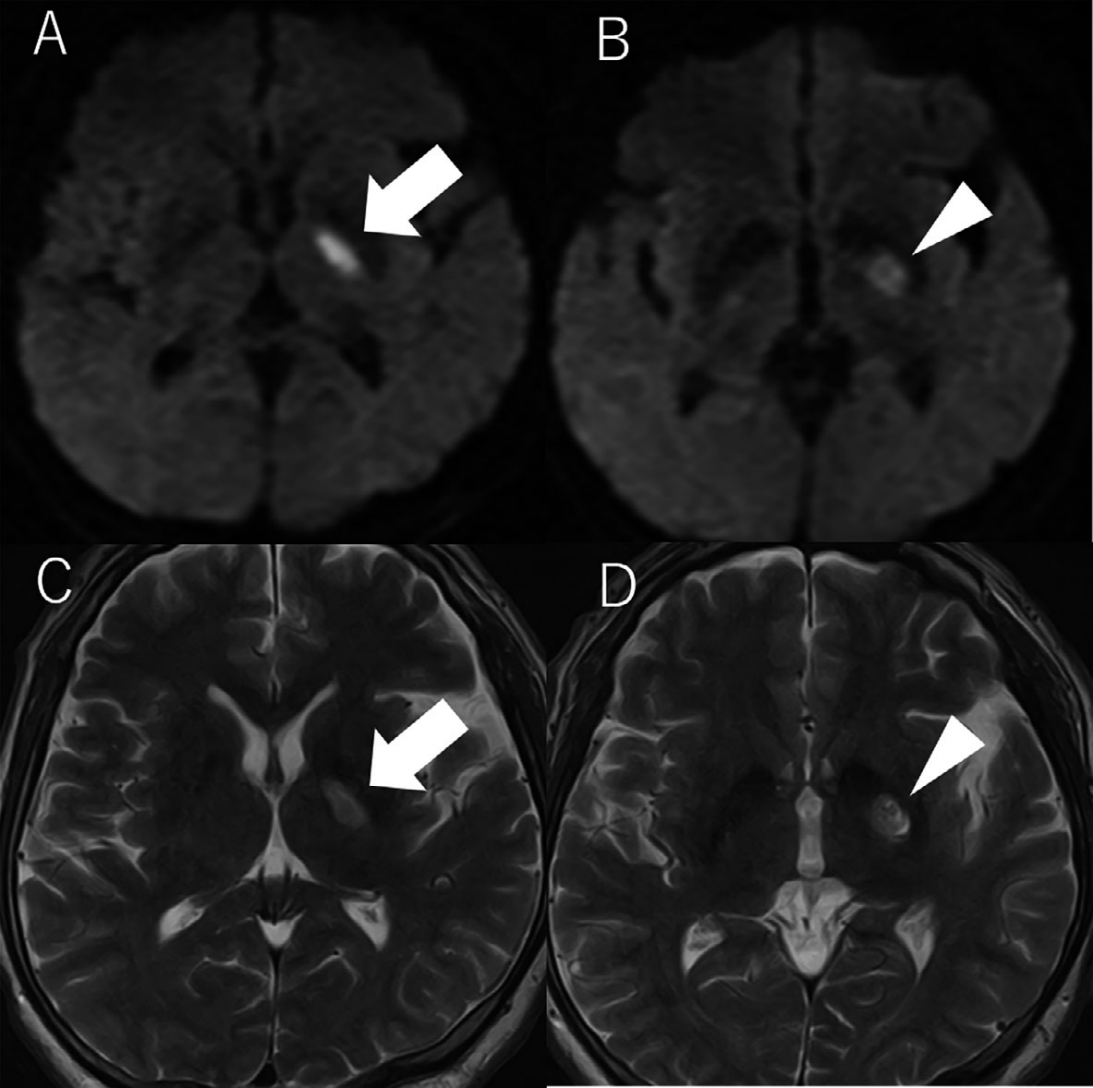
Diffusion‐weighted (A and B) and T2‐weighted (C and D) MRI images of cerebral infarction 1 month after pallidotomy. A and C show acute cerebral infarction on the left posterior limb of internal capsule (arrow). B and D show left pallidotomy lesion (arrowhead).
